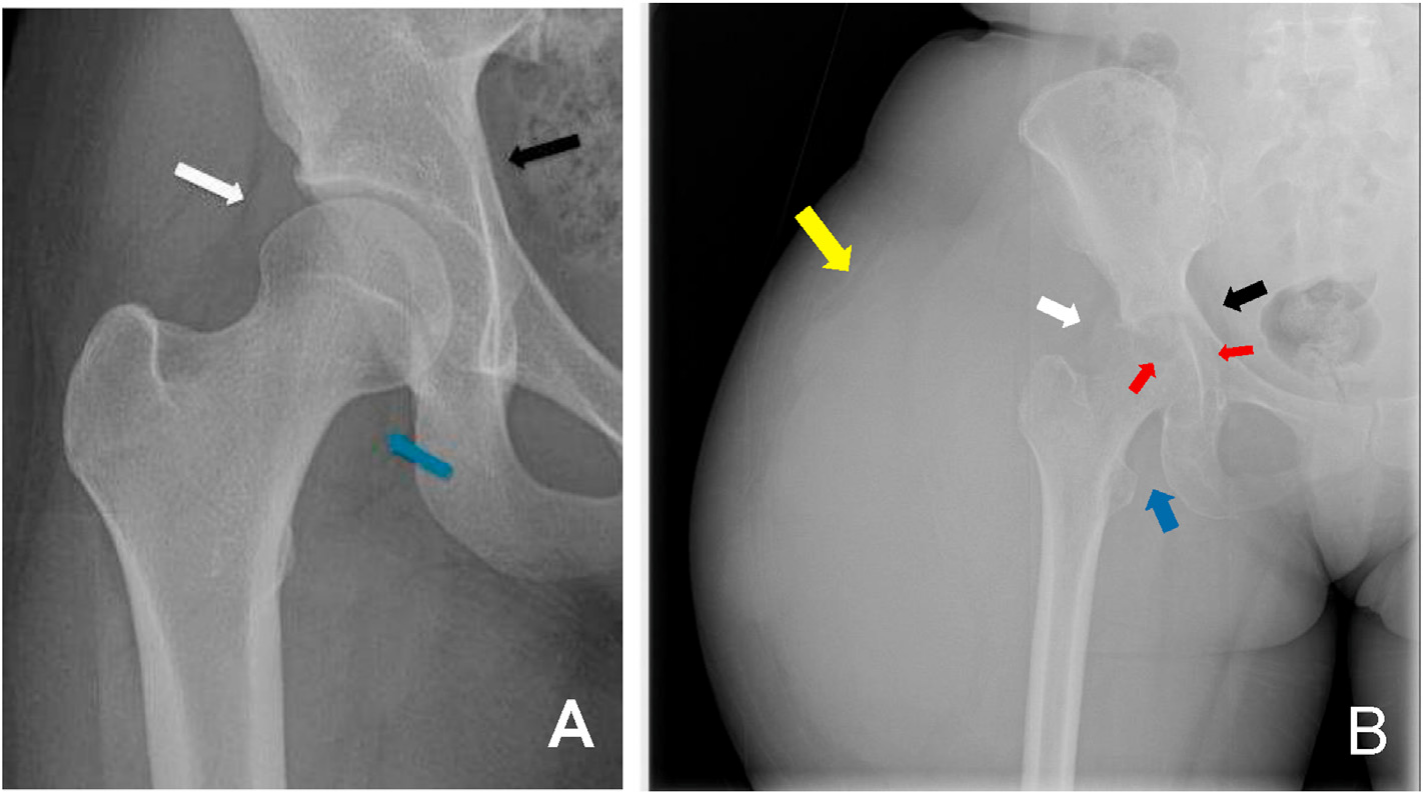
(A) Normal hip radiograph shows normal gluteal fat line (white arrow), iliopsoas fat line (blue arrow), and obturator fat line (black arrow). (B) The patient's right hip radiograph shows a slightly blurred gluteal fat line (white arrow) and obturator fat line (black arrow), with indistinct iliopsoas fat line (blue arrow). A large lobulated soft tissue mass at the lateral side of the femur (yellow arrow) with the destruction of femoral head and acetabular wall (red arrows), consistent with severe central erosion was observed.
